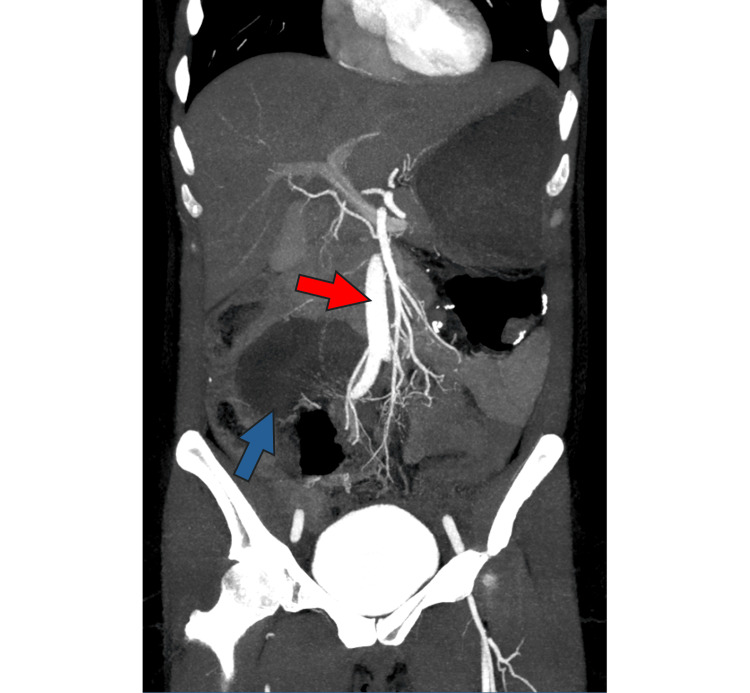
CT angiography of the abdomen and pelvis. As reported, the findings included the patient's abdominal aorta (red arrow) and its visceral branches, with no sign of arterial occlusion. Patent portal vein, mesenteric veins, inferior vena cava, and their major tributaries, no sign of thrombosis-occlusion. Some decreased enhancement in the walls of the distal ileal small bowel and mesenteric edema in the right lower quadrant noted (blue arrow), raising concern for small bowel ischemia.
